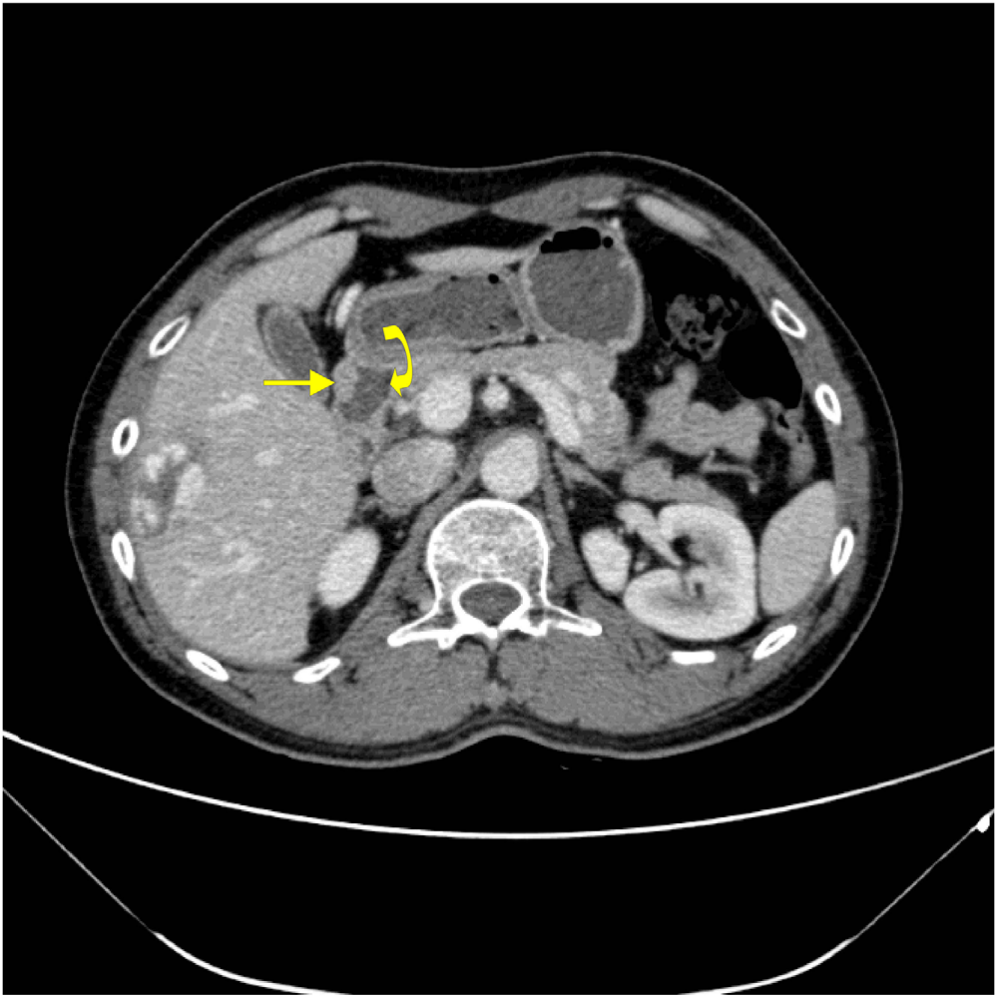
A 44‐year‐old man with hepatic carcinoma. Axial multiple detector spiral computed tomography (MDCT) arterial phase shows pancreatic tissue (arrow) surrounding the posterior wall of the duodena bulb (curved arrow)
